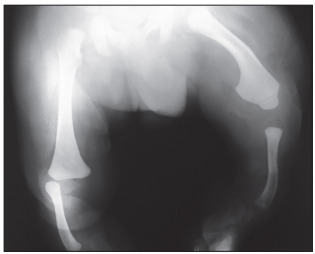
X-ray showing bilateral absence of the tibia with intact fibulae and distally hypoplastic left femur plus normal right femur.
